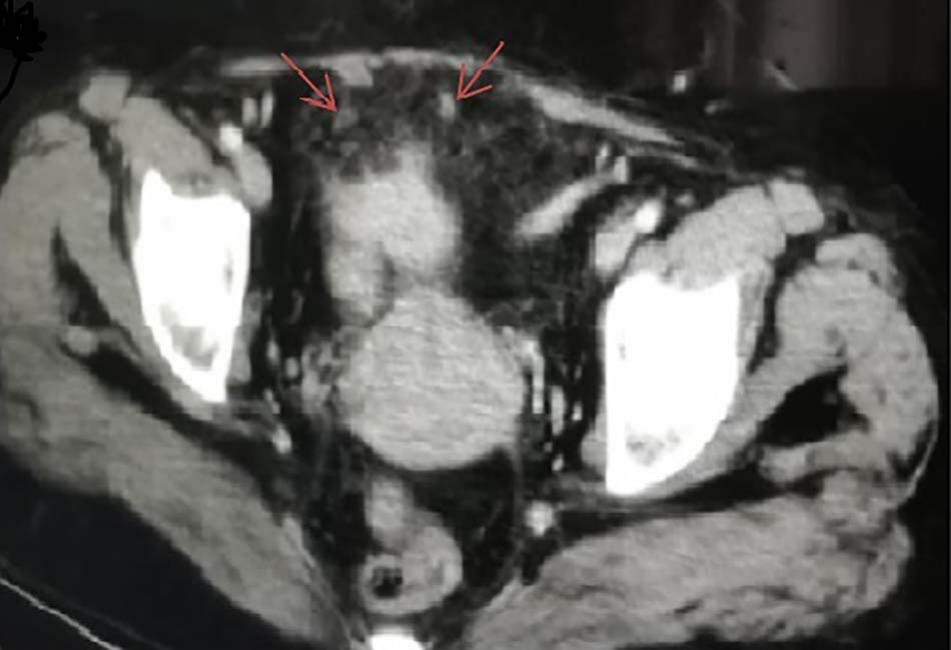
CT scan of abdomen and pelvis axial view showing intra-abdominal gallstones.
